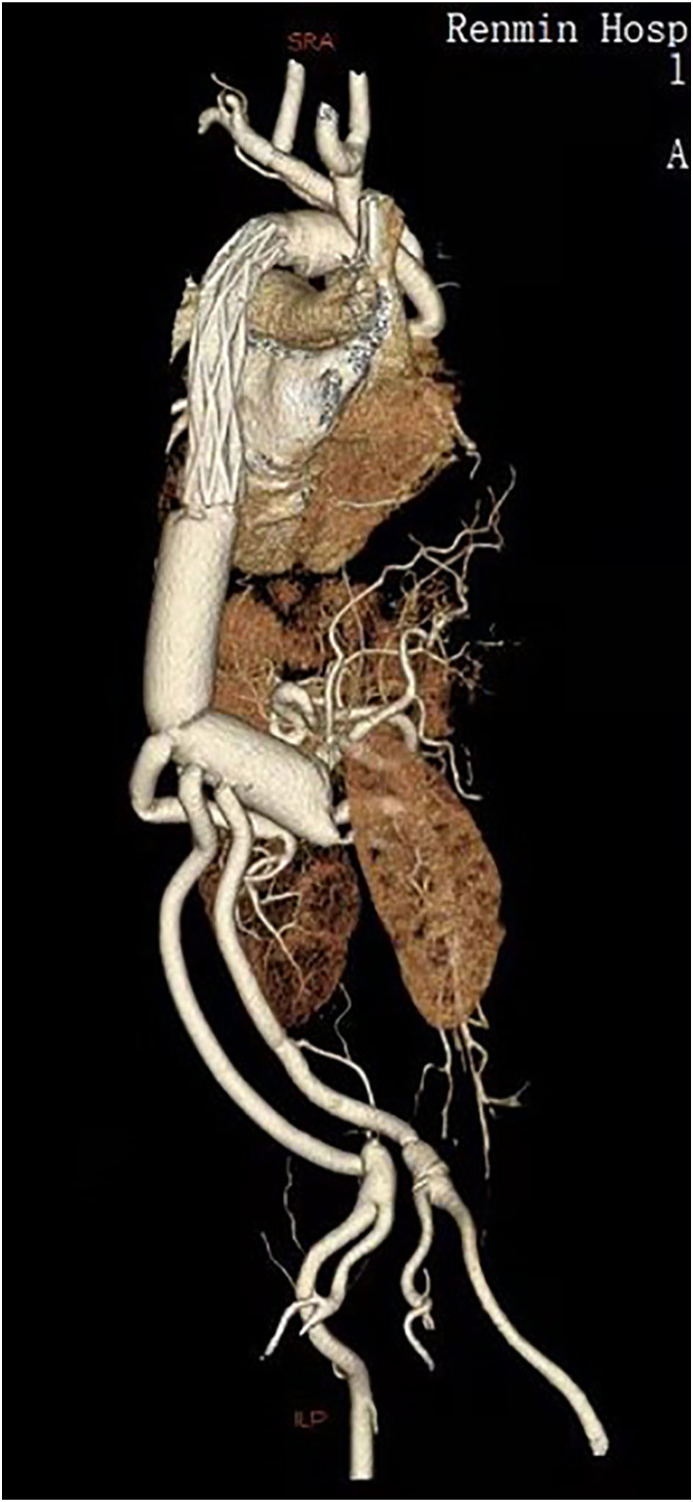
Post-operative CTA of the patient.
